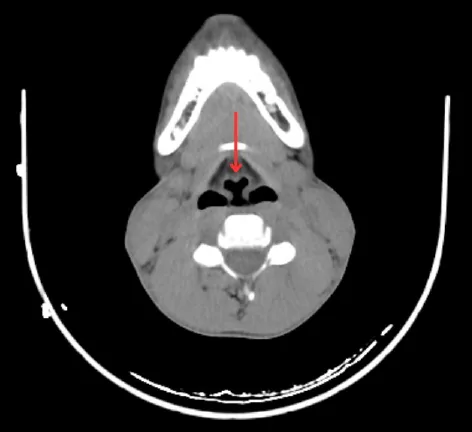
Non-contrast computed tomography of the neck at the level of the epiglottis obtained during inspiration, demonstrating abnormal inward prolapse of the mid-epiglottis (arrow) creating a heart-shaped airway configuration in an adolescent young patient who presented with sudden-onset respiratory distress and dyspnoea.
